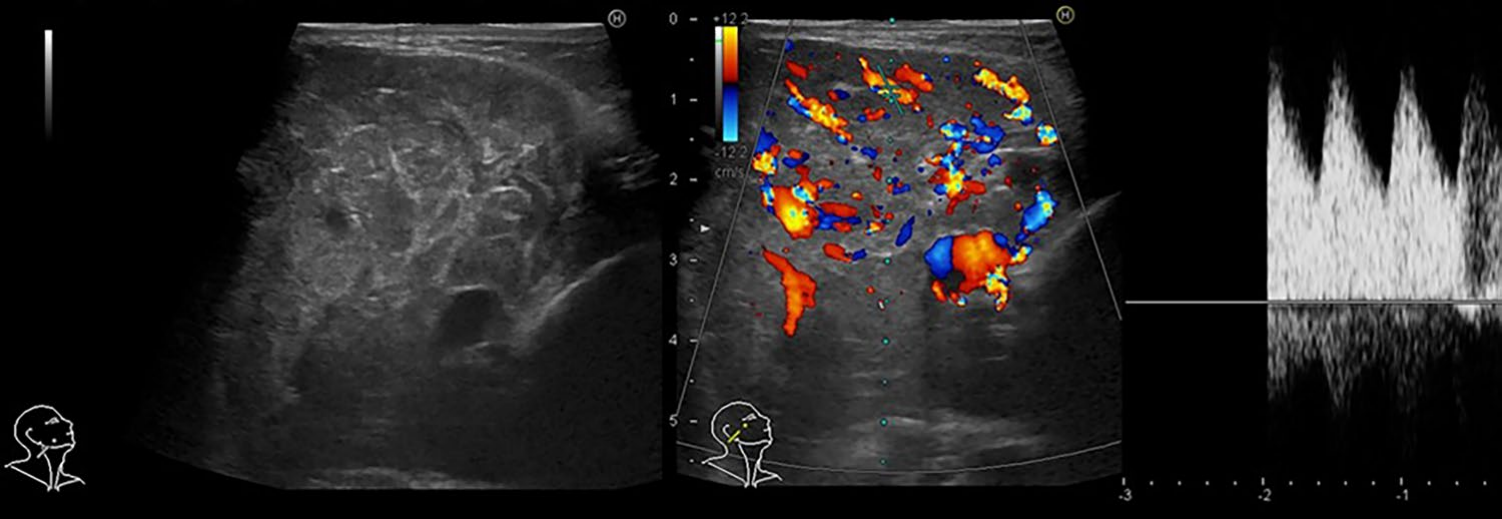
Four-month-old girl with infantile hemangioma. On ultrasonography, subcutaneous mass on right neck was revealed as a well-defined mass showing variable echogenicity and color Doppler shows arterial flow
